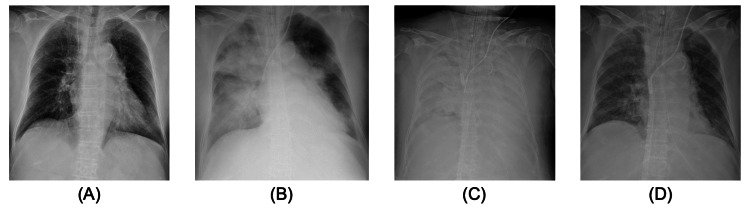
Chest X-ray findings during hospitalization Chest radiography did not reveal any abnormal findings on the day of admission (A). Ten hours after admission, chest radiography revealed bilateral diffuse alveolar opacities, suggesting acute respiratory distress syndrome (B). After starting venovenous extracorporeal membrane oxygenation, bilateral diffuse alveolar opacities deteriorated on the fourth day of hospitalization (C). On the 19th day of hospitalization, lung infiltration resolved, suggesting improvement in acute respiratory distress syndrome (D).
